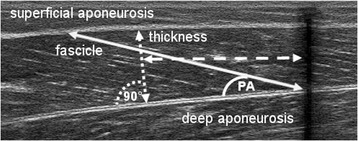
Ultrasound image of the GM muscle showing determination of muscle thickness (dotted line), fascicle length (solid line), and pennation angle (PA). Muscle thickness was measured at 50% of the muscle belly length (dashed line) determined from the echo of the applied tape (black shadow on the right side)
